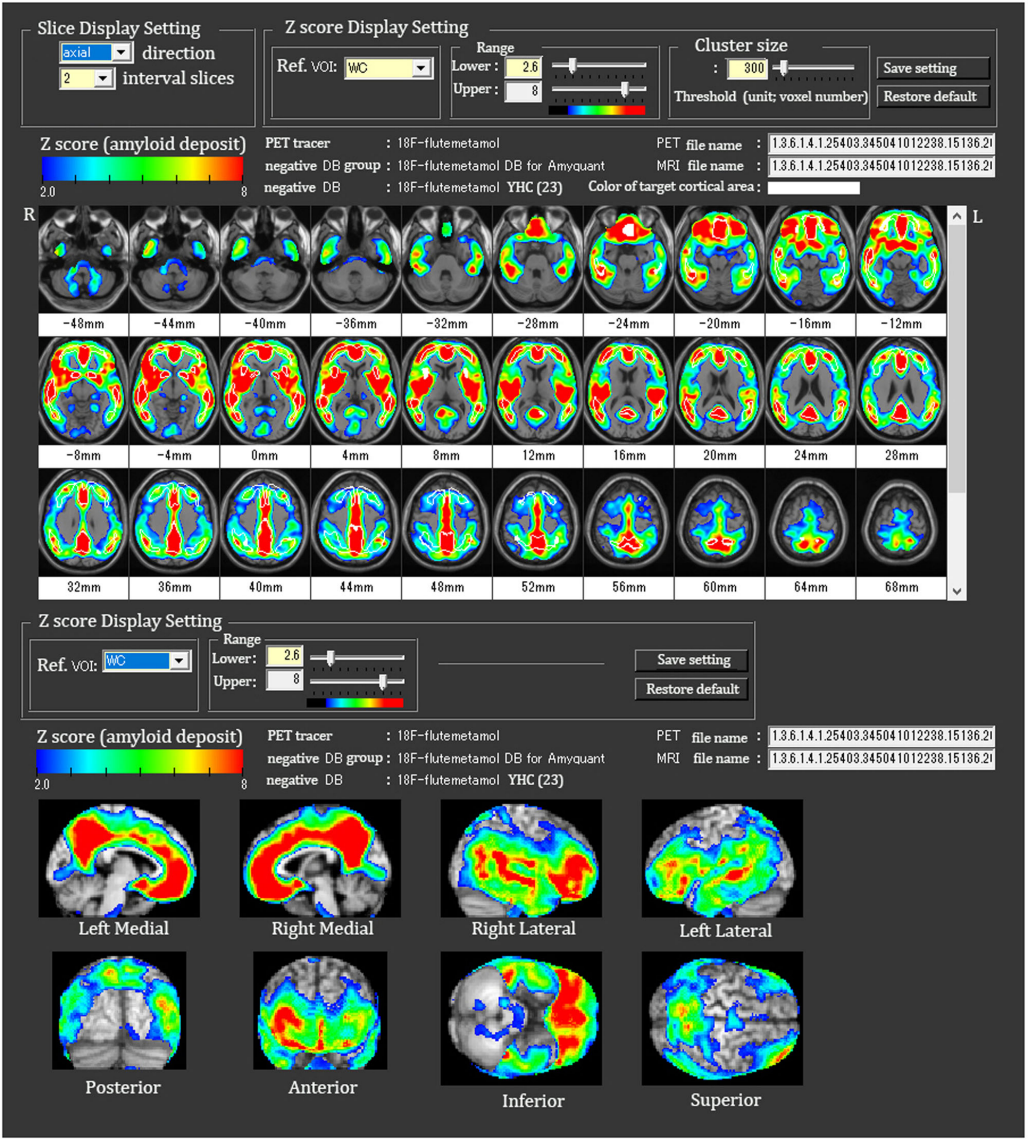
Z‐score mapping on tomographic sections and surface rendering of the standardized brain MRI. The target cortical and striatal areas are contoured by white lines. The lower and upper levels of the Z‐score can be changed along with a threshold of a cluster size
